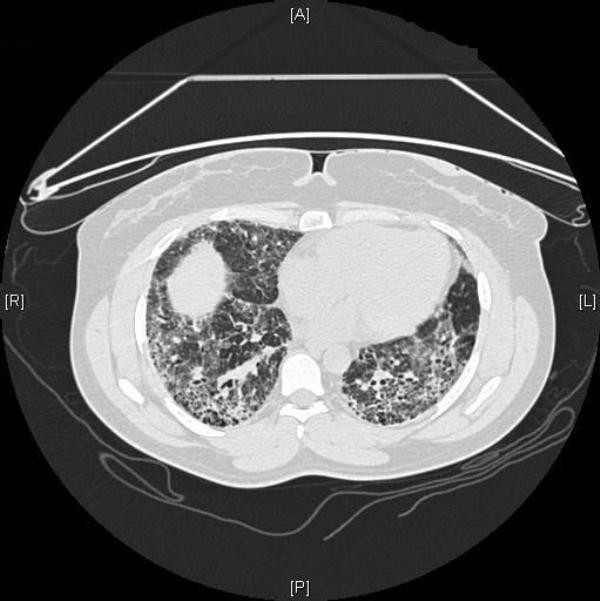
Case 1. High-resolution computed tomography (HRCT) scan at the basal portion of the lungs demonstrating ground-glass attenuation, reticular opacities, traction bronchiectasis, with honeycombing throughout both lungs.
